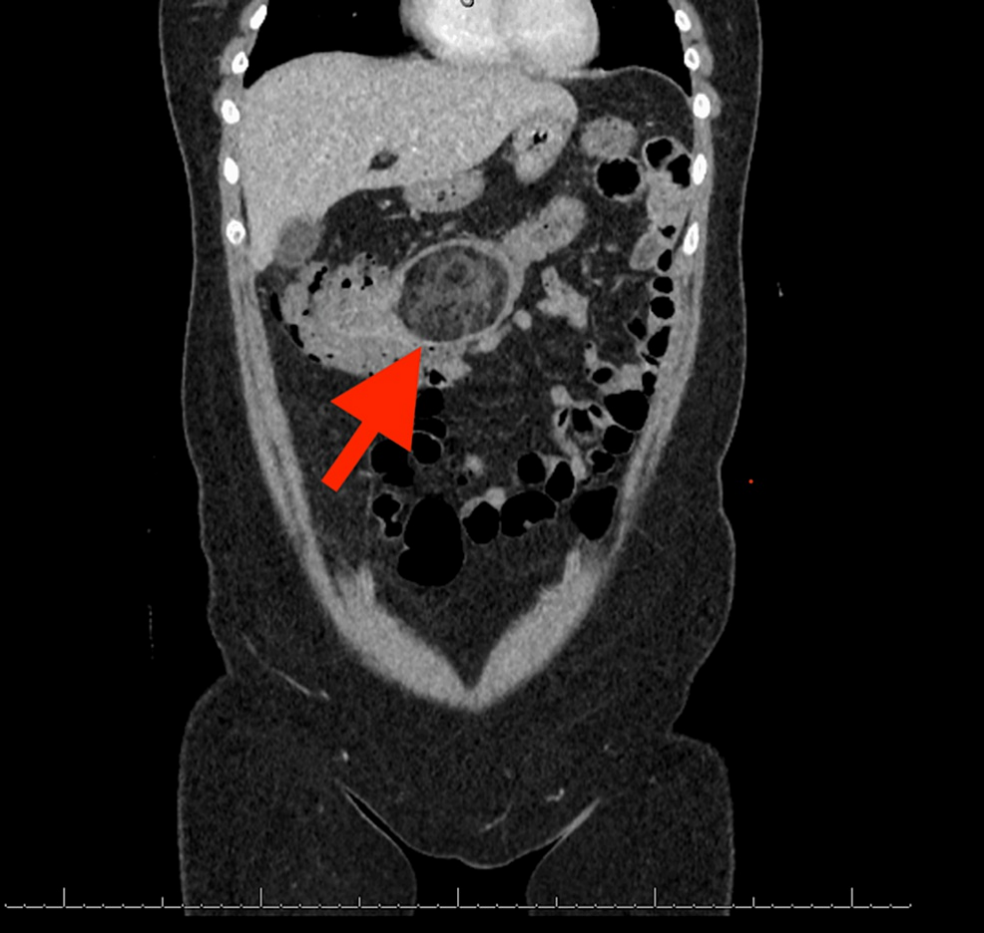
Contrast-enhancing CT coronal view demonstrating lipoma as the lead point for ileocolic intussusception (red arrow) CT: computed tomography
